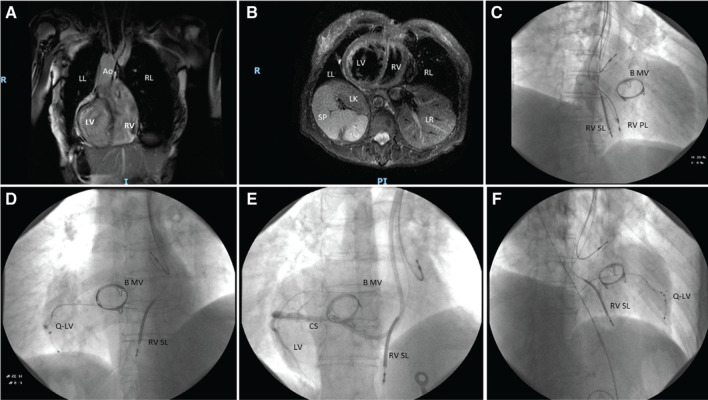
A: Cardiac MRI scan with long-axis view. B: Cardiac MRI scan in the short-axis view at papillary muscle sectioning. C: Anteroposterior view: dual-chamber pacemaker. D: CRT-D during “non-normalized” right anterior oblique view. E: Anteroposterior view coronary sinus during angiography. F: CRT-D during “normalized” left anterior view. B MV: biologic prosthetic mitral valve; CS: coronary sinus angiography; LL: left lung (bilobed lung); LR: liver; LV: left ventricle; Q-LV: quadripolar catheter in the left lateral vein; RK: right kidney; RL: right lung (trilobed lung); RV: right ventricle; RV PL: right ventricular pacing lead; RV SL: right ventricular shock lead; SP: spleen.
